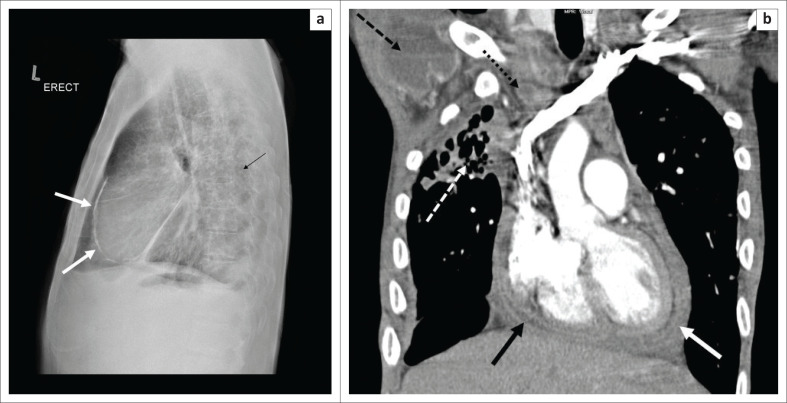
(a) A 68-year-old human immunodeficiency virus-negative male presented with features of pericarditis. GeneXpert and bronchial washings confirmed tuberculosis. A lateral chest radiograph demonstrates pericardial calcification (solid white arrows) and multiple pulmonary nodules (black arrow). (b) A 37-year-old male, human immunodeficiency virus positive with pulmonary and pericardial tuberculosis, confirmed with GeneXpert on both sputum and pericardial fluid. Coronal post-contrasted computed tomography of the chest demonstrates pericardial thickening (solid white arrow), small pericardial effusion (black arrow), large necrotic right axillary (black arrow with dashed line), smaller necrotic mediastinal (black arrow with dotted line) lymph nodes and right apical post-infective bronchiectasis (white arrow with dashed line).
